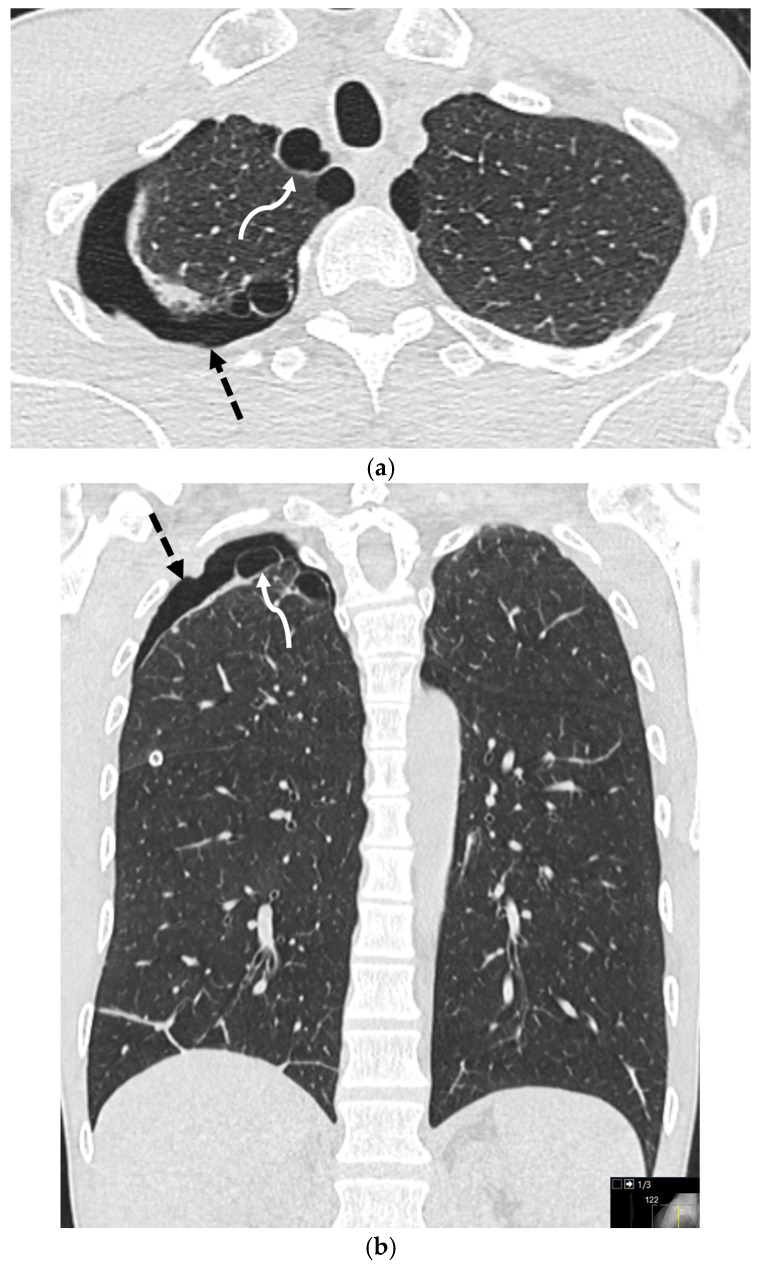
45-year-old male with no known past medical history presented to the emergency department with right-sided chest pain and dyspnea. He was found to have a large right-sided pneumothorax. Axial (a) and coronal (b) images from CT chest in lung windows demonstrate a small right pneumothorax (dashed arrows). Incidental note is made of apical pleural blebs (curved arrows).
